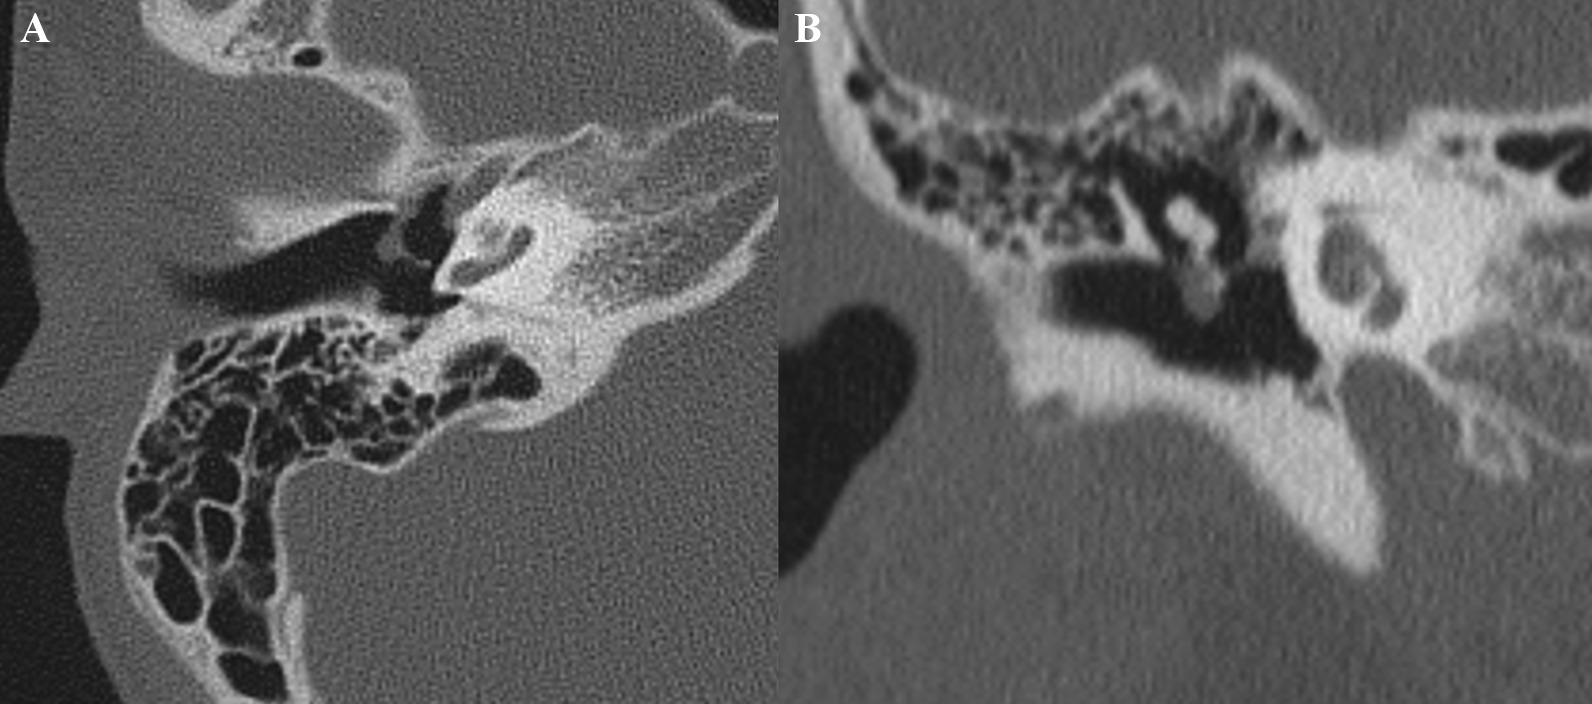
Temporal bone computed tomography revealing a 0.3 × 0.3-cm soft tissue density, cystic lesion in the right tympanic membrane, abutting on the malleus (A axial view, B coronal view)
